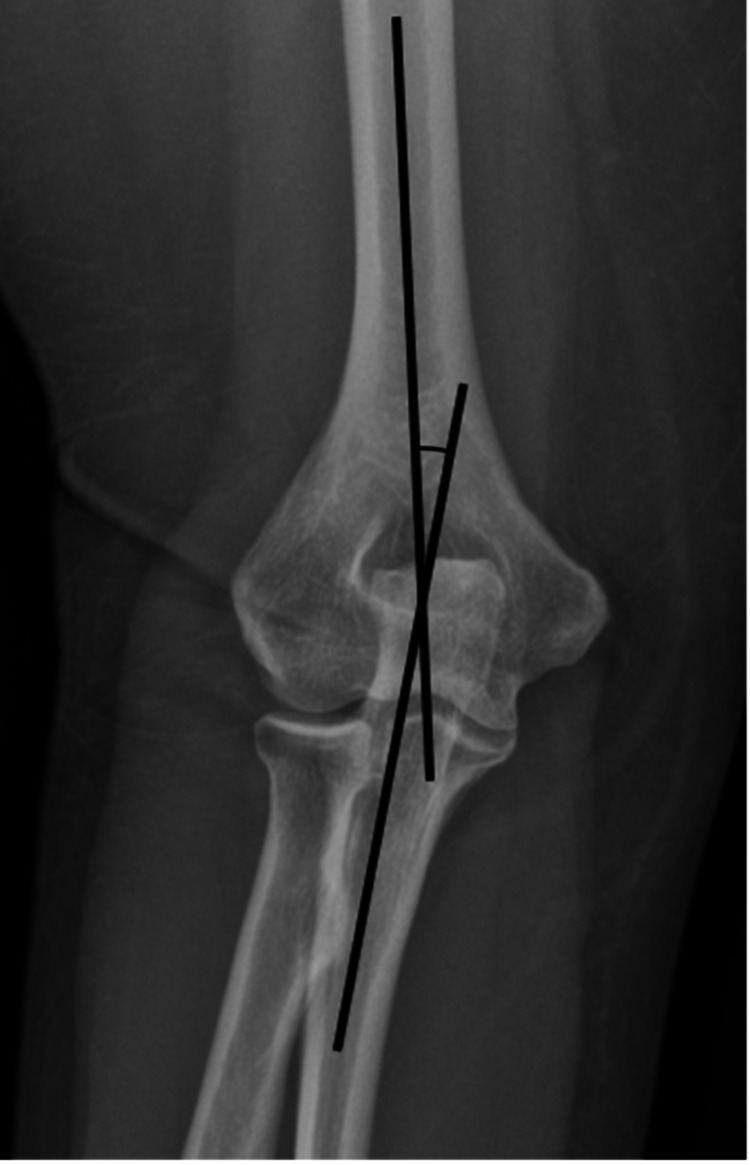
Measurement of the elbow carrying angle on anteroposterior elbow radiographs.
